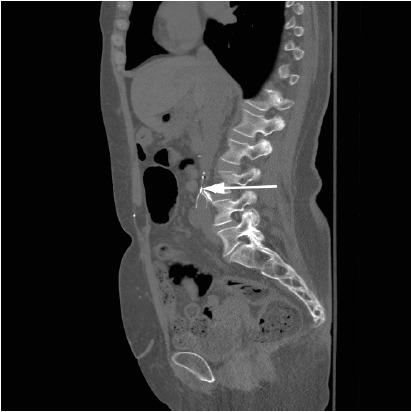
Sagittal computed tomography view of lumbosacral spine. Arrowhead demonstrates fracture and displacement of the posterolateral inferior vena cava filter leg.
